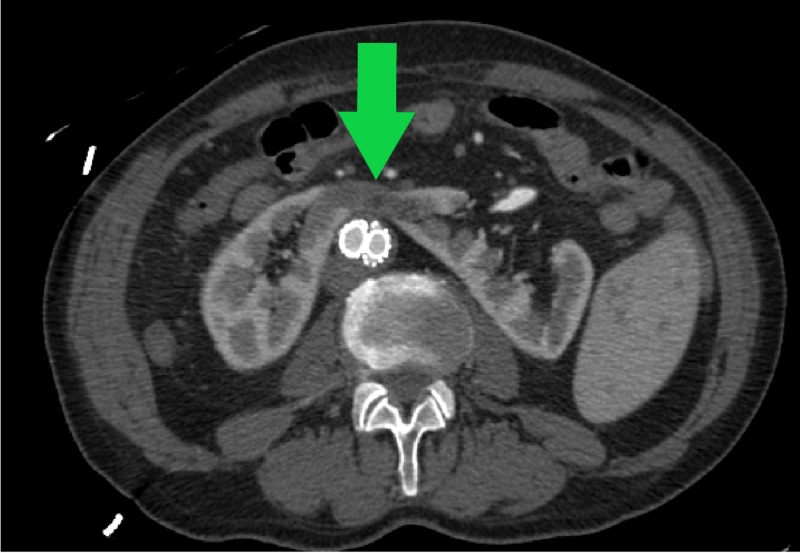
Postoperative abdominal CT angiogram demonstrating hypodense, infarcted HSK isthmus (green arrow) HSK, horseshoe kidney
